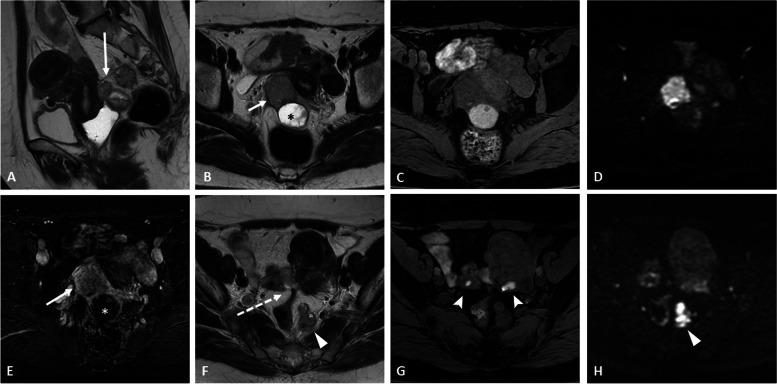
Endometrioid adenocarcinoma developed on deep pelvic endometriosis in a 58-year-old woman with a history of infertility. Sagittal (a) and axial (b) T2-weighted images show a large heterogeneous mass obliterating the Douglas cul-de-sac composed of solid (white arrows) and cystic (asterisk) portions. Axial fat-suppressed T1-weighted image shows hemorrhagic content within the cystic cavity (c). Diffusion-weighted imaging (d high *b* value) shows a high signal intensity within the solid portion with corresponding enhancement on axial enhanced fat-suppressed T1 image (e). Axial T2-weighted sequence at a higher level (f) shows the features of deep pelvic endometriosis of the posterior compartment (dotted arrow) and a metastatic nodule of the mesorectum (arrowhead). “Kissing ovaries” containing small-sized endometrioma (arrowheads) are seen on corresponding fat-suppressed T1-weighted image (arrowheads (g)). Restricted diffusion within the mesorectum metastasis is also seen (h)
